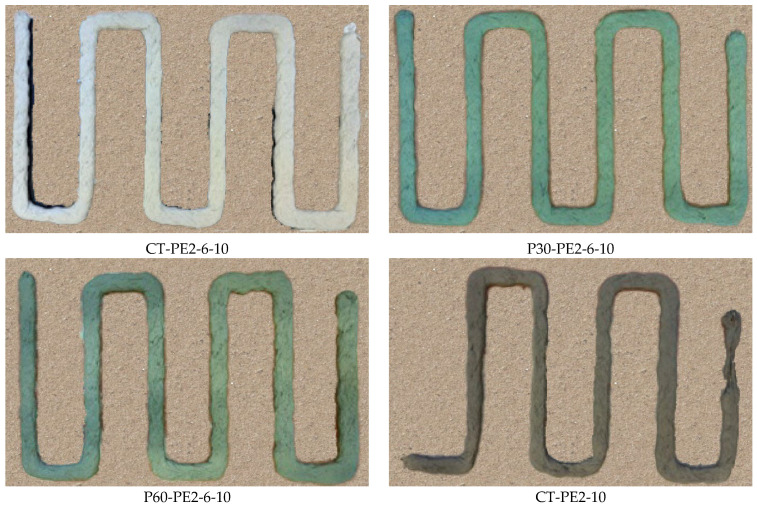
Extrudability of ECC mixes CT-PE2-6-10, P30-PE2-6-10, P60-PE2-6-10, and CT-PE2-10.
